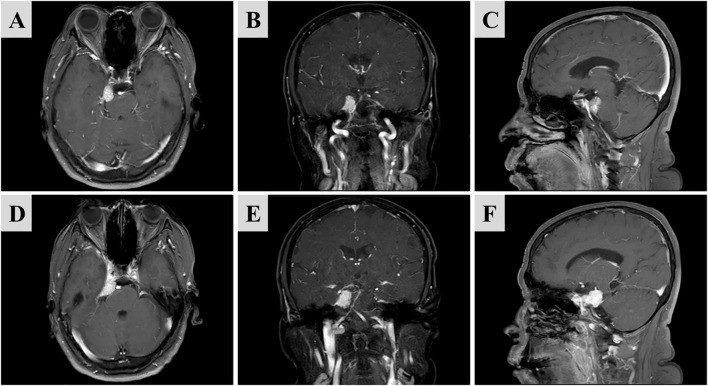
Preoperative contrast-enhanced MRI demonstrating a mass in the right cerebellopontine angle. Homogeneous enhancement is visible at the right petrous apex. The dural tail sign could be seen on the axial plane. (A, D) Axial; (B, E) Coronal; (C, F) Sagittal.
